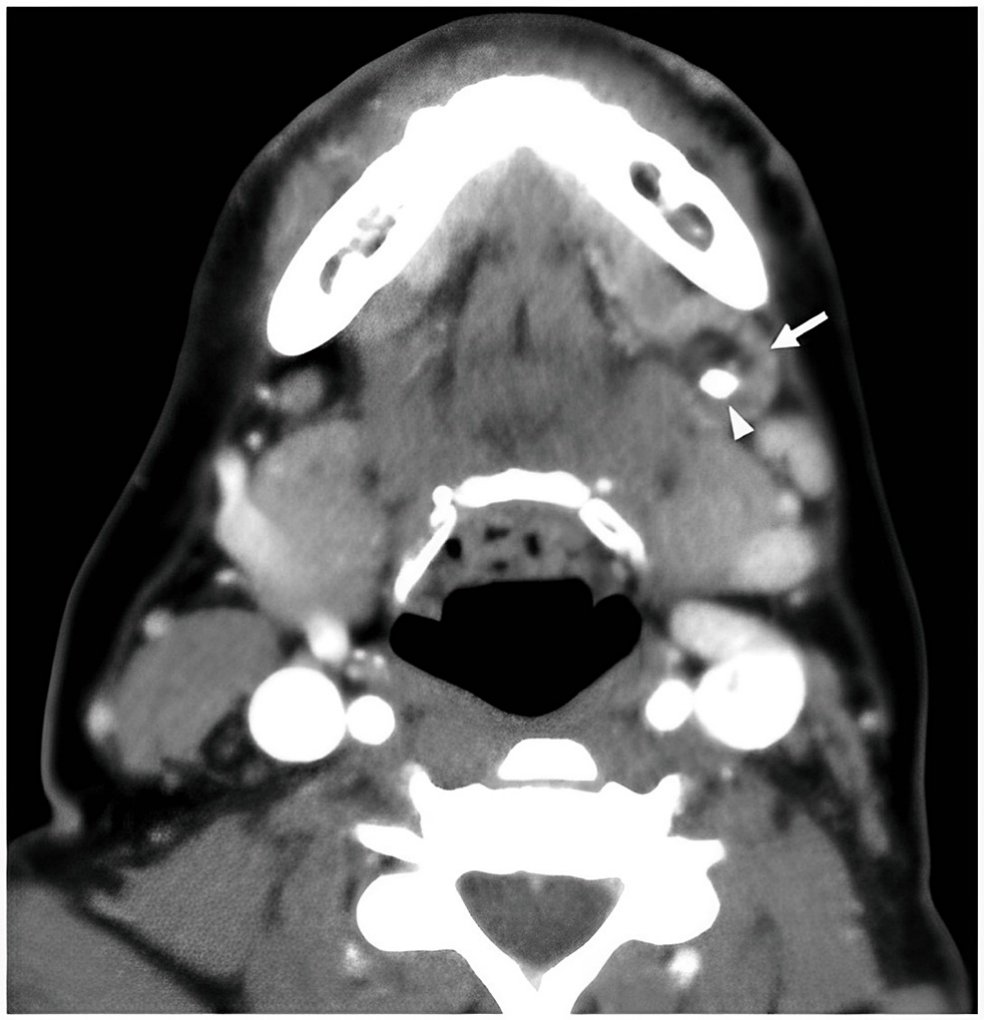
CT scan neck showing the sialolith
